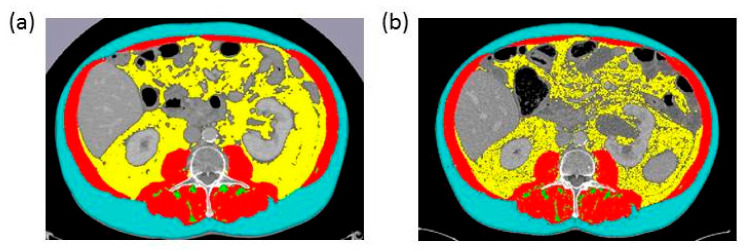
Axial CT images of the third lumbar vertebra region (a) before the initiation of FLOT chemotherapy and (b) after 4 cycles of FLOT chemotherapy in the same patient. In red, lumbar skeletal muscle area (SMA) (cm^2^); in yellow, visceral adipose tissue area (VAT) (cm^2^), in turquoise: subcutaneous adipose tissue area (SAT) (cm^2^); in green, intramuscular adipose tissue area (IMAT) (cm^2^).
